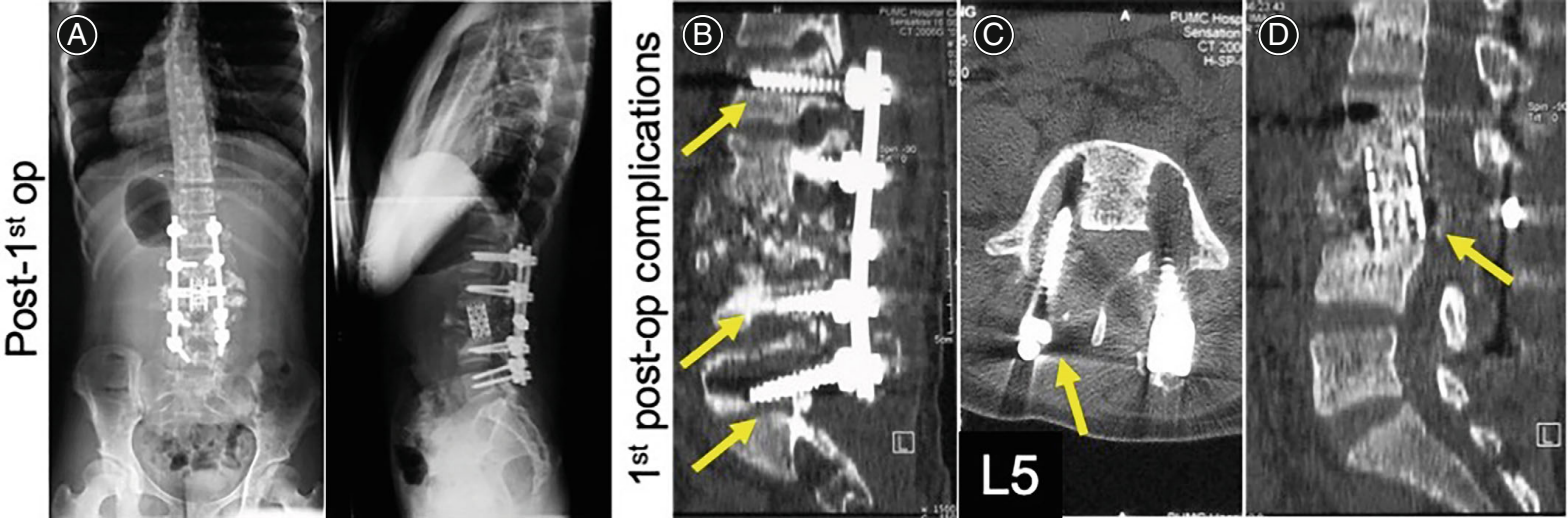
(A) Radiology, 3 months after the primary surgery: L2 and L3 corpectomy, instrumentation from T12 to L5, mesh cage. (B) Six months after the primary surgery, loosening pedicle screws of L1, L4, and L5. (C) MRI shows the loosening pedicle screws of L5. (D) Displaced mesh cage. Figure 2A is cited from our previously published report on this case.^5^
